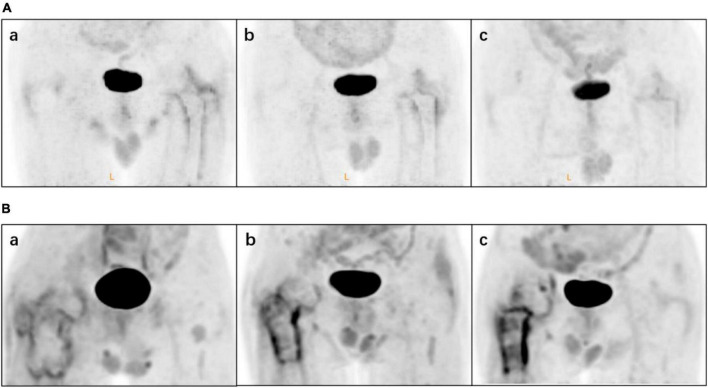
Two patients had multiple ^18^F-fluorodeoxyglucose (^18^F-FDG) positron emission tomography (PET)/computed tomography (CT) images. Patient (A) underwent total hip arthroplasty (THA) during chemotherapy: (a) 6 months, (b) 18 months and (c) 31 months postoperatively. Patient (B) with a tumor prosthesis underwent ^18^F-FDG at (a) 1 month, (b) 5 months and (c) 12 months postoperatively.
